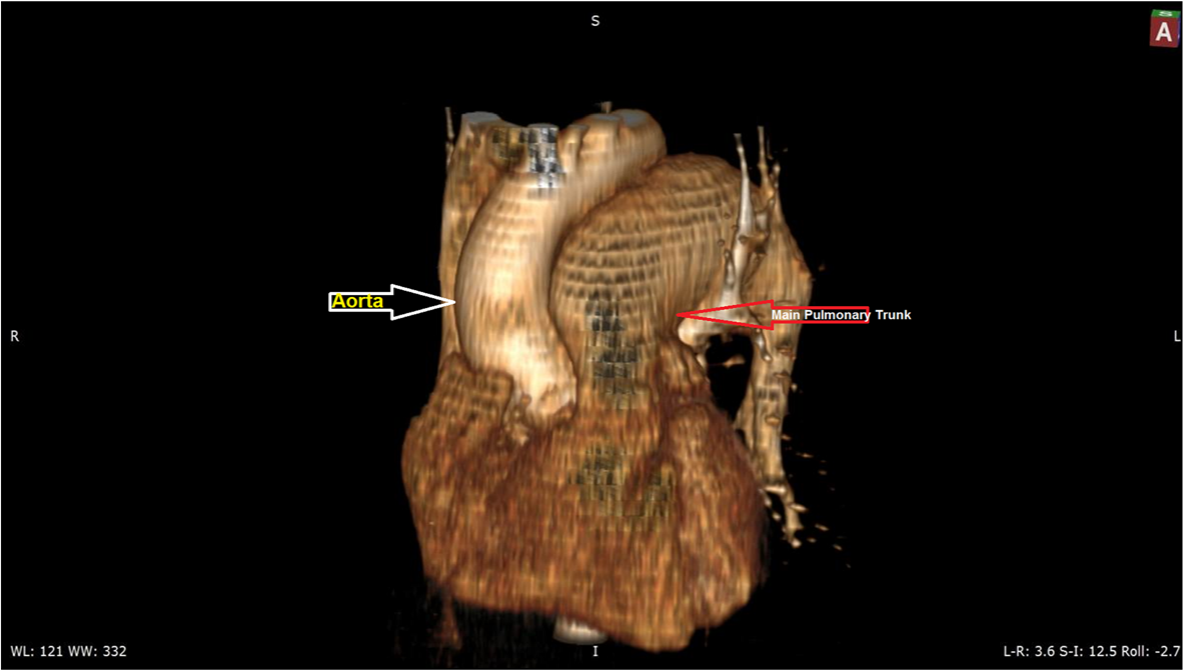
3D - Chest computed tomography angiogram scan (CT) showing: Hugely dilated pulmonary trunk compressing the adjacent Aorta
